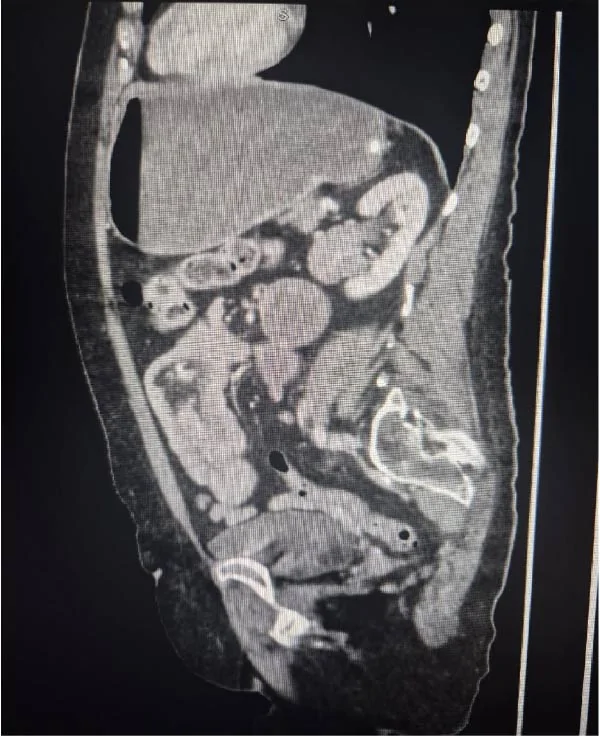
Sagittal view of emergency CT abdomen pelvis demonstrating intussusception as well as air fluid level with a distended stomach.
